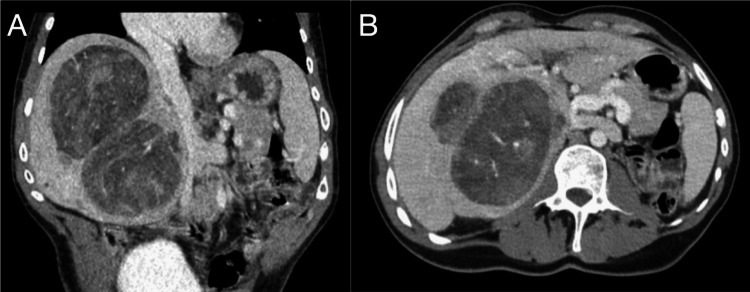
Pre-transplantation CT demonstrating hepatic lesions and type 1B Abernathy syndrome (A-B) Two dominant lesions noted in the left liver, measuring 9.3 x 8.6 cm and 10.8 x 7.9 cm. Several smaller lesions were also noted in the left liver. Delayed images demonstrated portal vein (PV) anatomy. Direct drainage of the PV into the infra-hepatic inferior vena cava (IVC) was noted in (A) axial and (B) coronal views.
